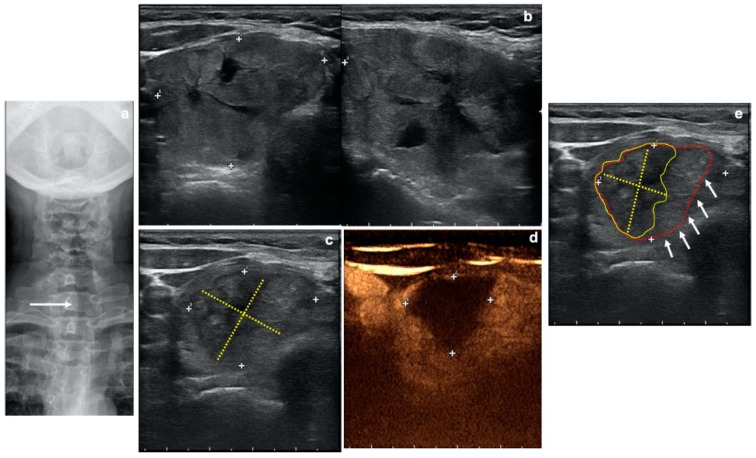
Case of a 56-year-old woman treated with radiofrequency ablation. *(*a) Thoracic inlet X-ray showing a large retrosternal goiter with left deviation (white arrow) and indentation of the trachea. *(*b) Two-planes gray-scale ultrasound (US) showing pretreatment evaluation of an almost completely solid right thyroid lobe nodule (Volume: 28.8 mL). *(*c) Gray-scale US at 6 months after treatment showing volumetric reduction of the ablated nodule (yellow dotted lines = evaluation of the ablated area with B-mode US). *(*d) Contrast-enhanced ultrasound (CEUS) at 6 months after treatment demonstrating the area of ablation as an area with lack of enhancement (white crosses = evaluation of the ablated area with CEUS). *(*e) After CEUS-treated nodule evaluation, treatment results can be better reconsidered on the grey-scale US (c) showing real ablated nodule margins (yellow dotted lines and encircled area) as compared to previously measured margins (white arrows; red encircled area).
